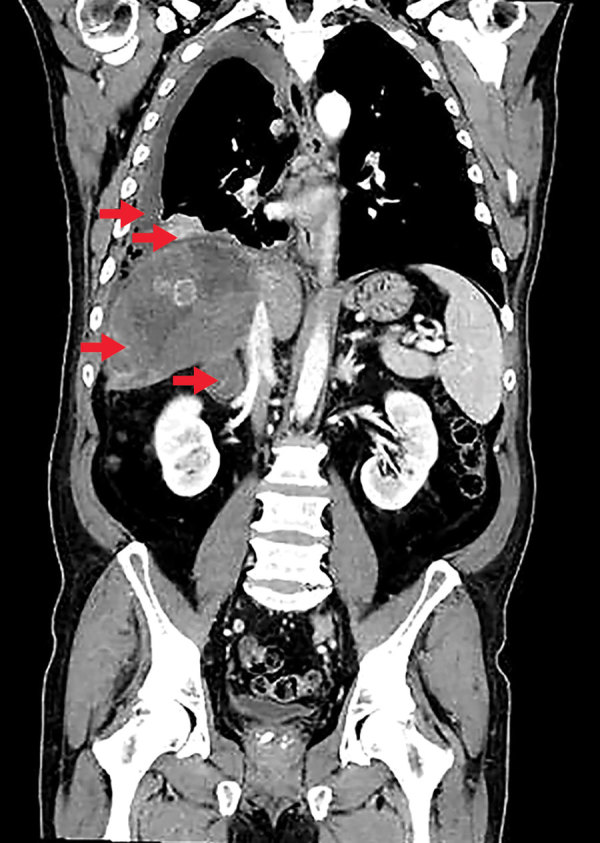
Computed tomography scan of a patient diagnosed with alveolar echinococcosis, Croatia. Arrows indicate right pleural effusion, lung lesions, an enlarged right adrenal gland, and a 13 × 12 × 12 cm lesion in the liver caused by *Echinococcus multilocaris*.
